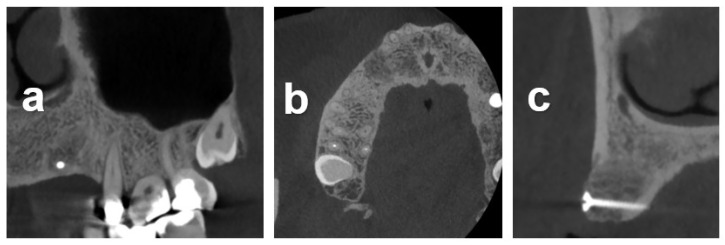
Sagittal (a), transversal (b), and frontal (c) 2D CBCT sections of the block-augmented area obtained 5 months after augmentation.
